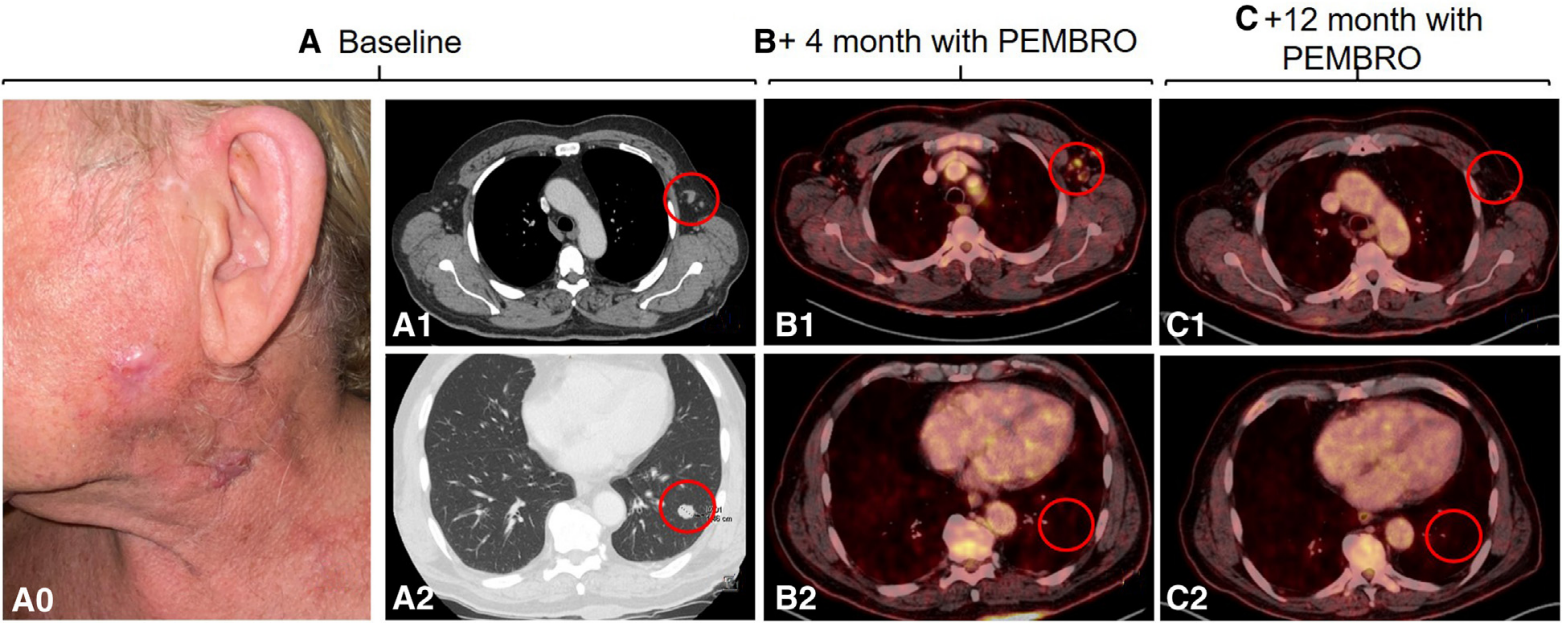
A, Baseline left cervical cutaneous squamous cell carcinoma. A0, Clinical image; A1, axillary lymph nodes by CT; A2, pulmonary metastases by CT. B, Dissociate response after 4 months of pembrolizumab treatment. B1, Lymph node pseudoprogression in PET/CT; B2, complete response of the lung metastases by PET/CT. C, Complete response after 12 months with pembrolizumab treatment. C1, Complete response of axillary lymph nodes by PET/CT; C2, complete maintained response of the lung metastases by PET/CT. *PET*, Positron emission tomography; *CT*, computed tomography.
